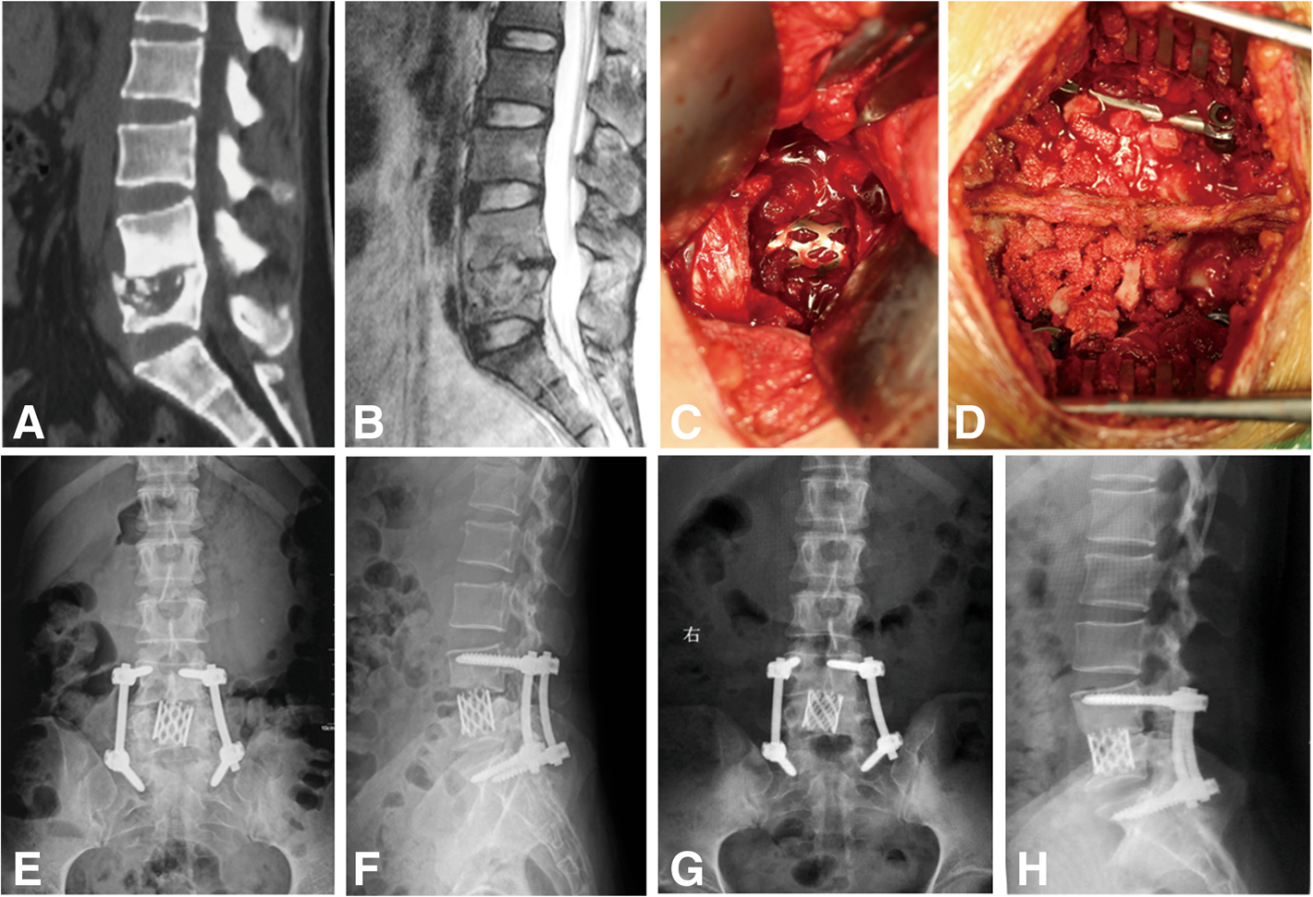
Twenty-seven-year-old woman with lumbosacral tuberculosis (L4-S1 level). a, b Preoperative computed tomography and magnetic resonance imaging scans demonstrate vertebral body destruction and abscess. c Anterior debridement and titanium cage strut fusion. d Posterior internal fixation and graft fusion. e, f Postoperative radiographs show well-positioned internal fixation. g, h Radiographs show satisfactory focal clearance and strut graft stability at the final follow-up
